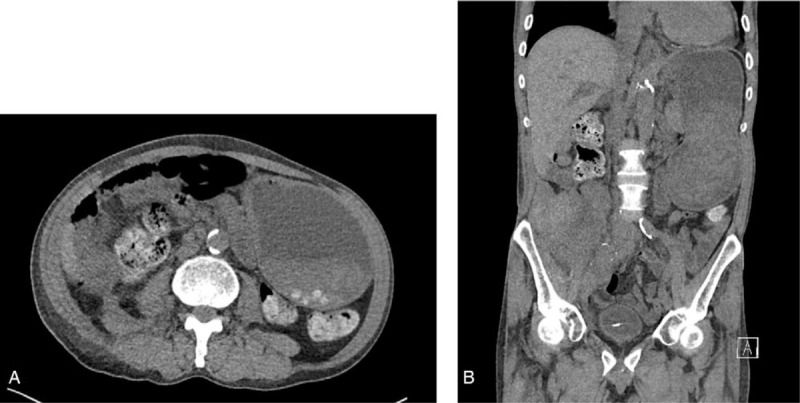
Computed tomography. There was no structural abnormality other than gastric distention.
